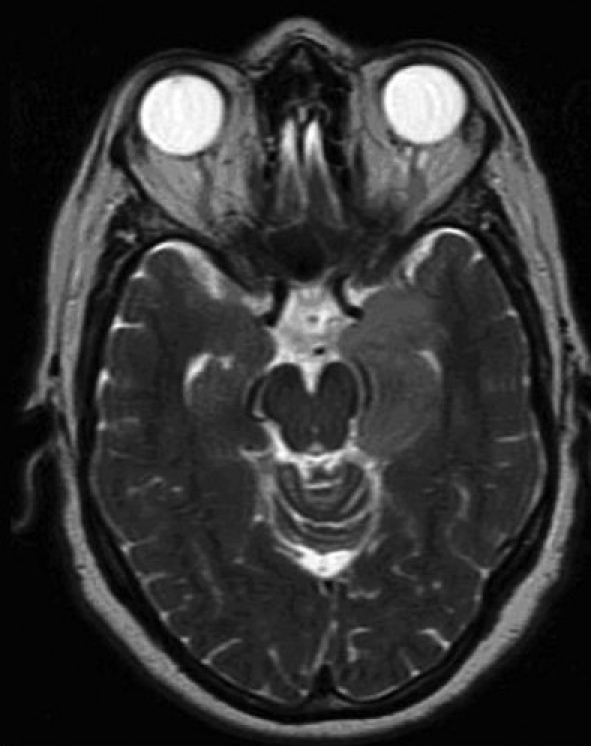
T2-weighted MR images without gadolinium. Left temporal lobe tumor with hyperintense signal
